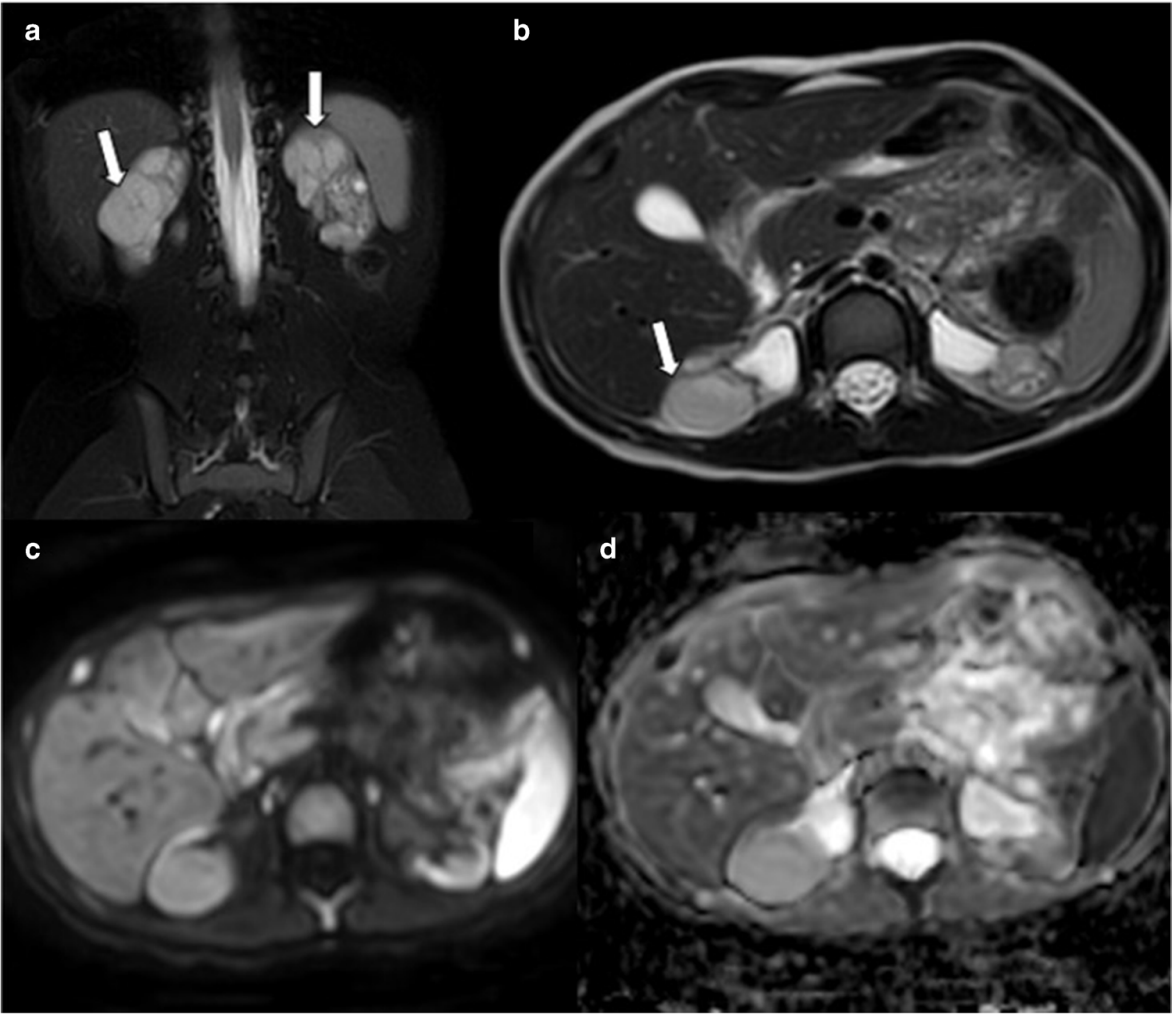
Scarred kidney and regeneration nodules. A 6-year-old boy undergoing ultrasound follow-up for chronic kidney disease was found to have multiple lesions in both kidneys. a, b Coronal fat-saturated (a) and axial non-fat-saturated (b) T2-weighted images show multiple bilateral hyperintense nodular lesions (*arrows*) located between scarred atrophic renal tissues. Note that these lesions have homogeneous signal intensity. c, d Diffusion-weighted imaging (*b* = 600 s/mm^2^) (c) and apparent diffusion coefficient map (d) demonstrate no restricted diffusion in the lesions, consistent with regeneration nodules
